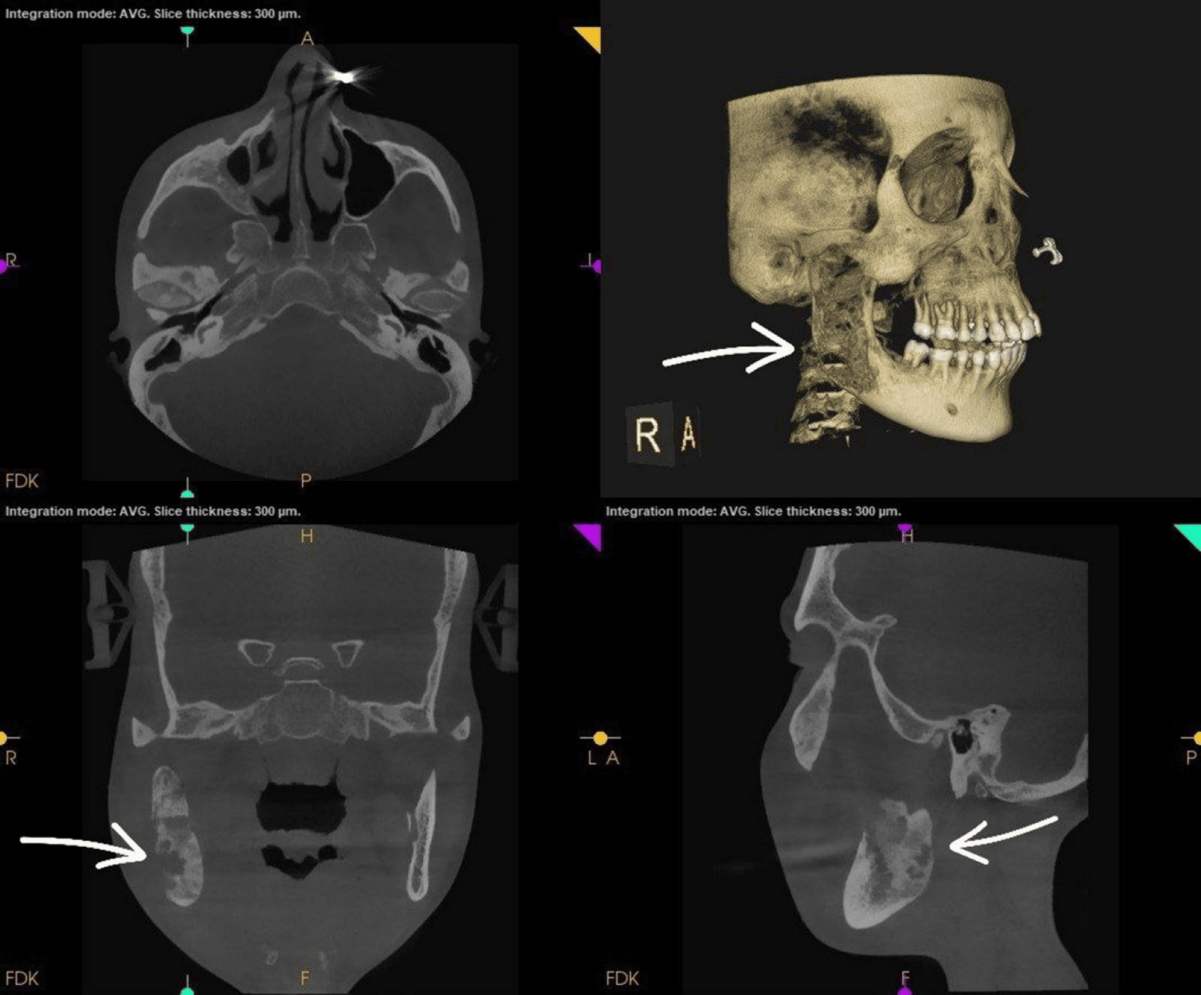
Pre-operative CT scan showing bony changes in the ramus on the right side of the mandible CT - Computed Tomography
